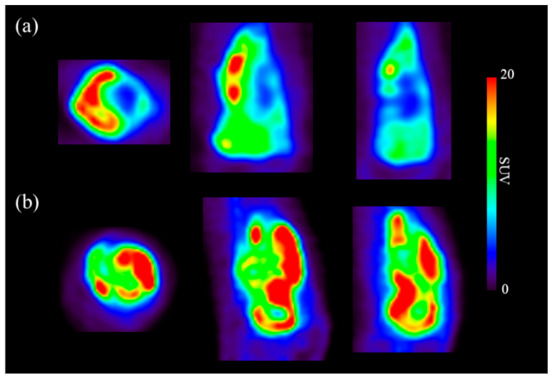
Transverse, coronal, and sagittal sections from osteosarcoma ^18^F-FDG PET/CT images. (a) Images from a chemotherapy responder; (b) images from a chemotherapy non-responder.
